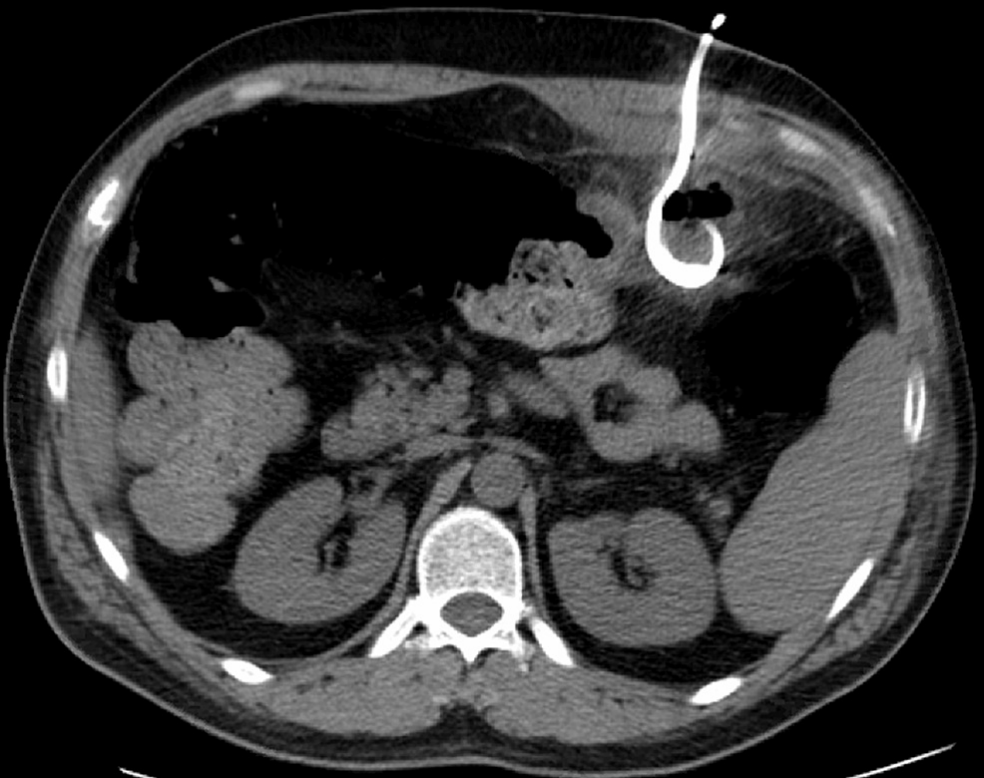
Image-guided CT peritoneal catheter drainage placement within the fluid collection. CT, computerized tomography
